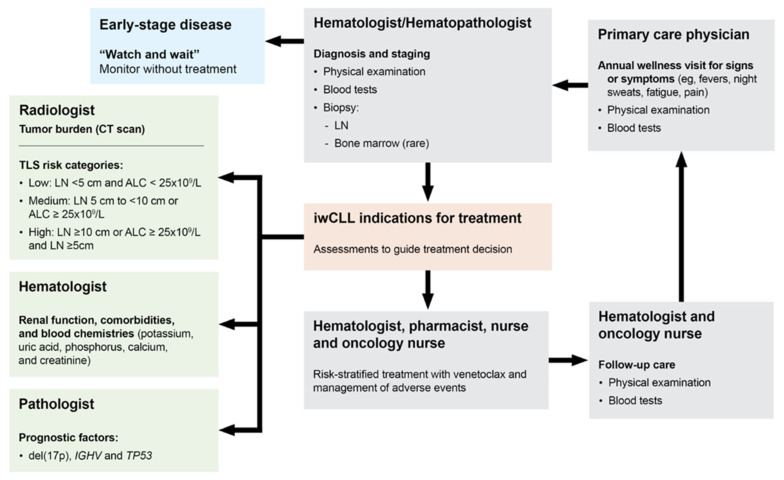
The journey of patients with CLL involves the coordination of a multidisciplinary team and shared decision-making. ALC, absolute lymphocyte count; CT, computerized tomography; del(17)p, deletion of chromosome 17p; IGHV, immunoglobulin heavy variable; iwCLL, International Workshop on Chronic Lymphocytic Leukemia; LN, lymph node; TLS, tumor lysis syndrome; TP53, tumor protein 53.
